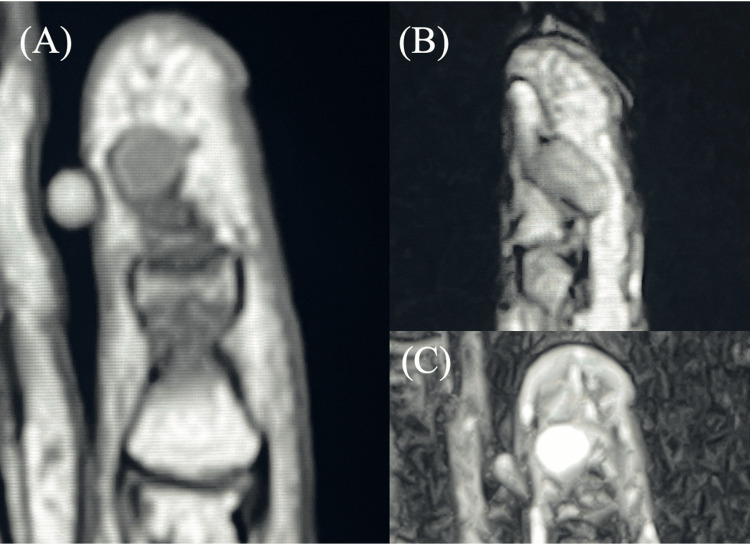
Preoperative MRI findings for case 6. A tumorous lesion that compresses the distal phalanx of the fingertip exists. (A) Low signal on a coronal T1-weighted image. (B) Isointense signal on a sagittal T2-weighted image. (C) High signal intensity on an axial STIR image. STIR: Short tau inversion recovery
